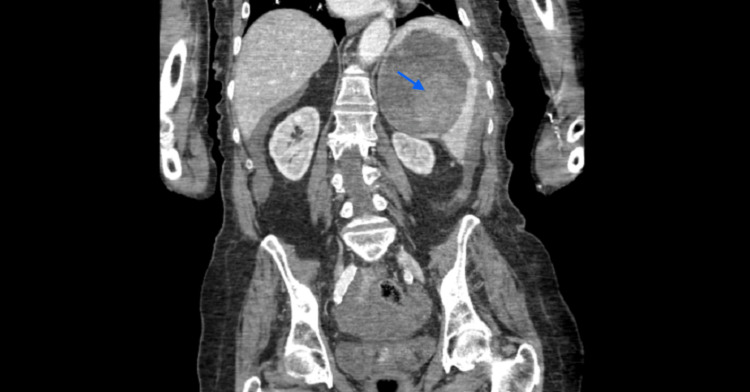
Coronal abdominal CT imaging showing a ruptured splenic hemangioma. Splenic lesion Identified by a blue arrow.
